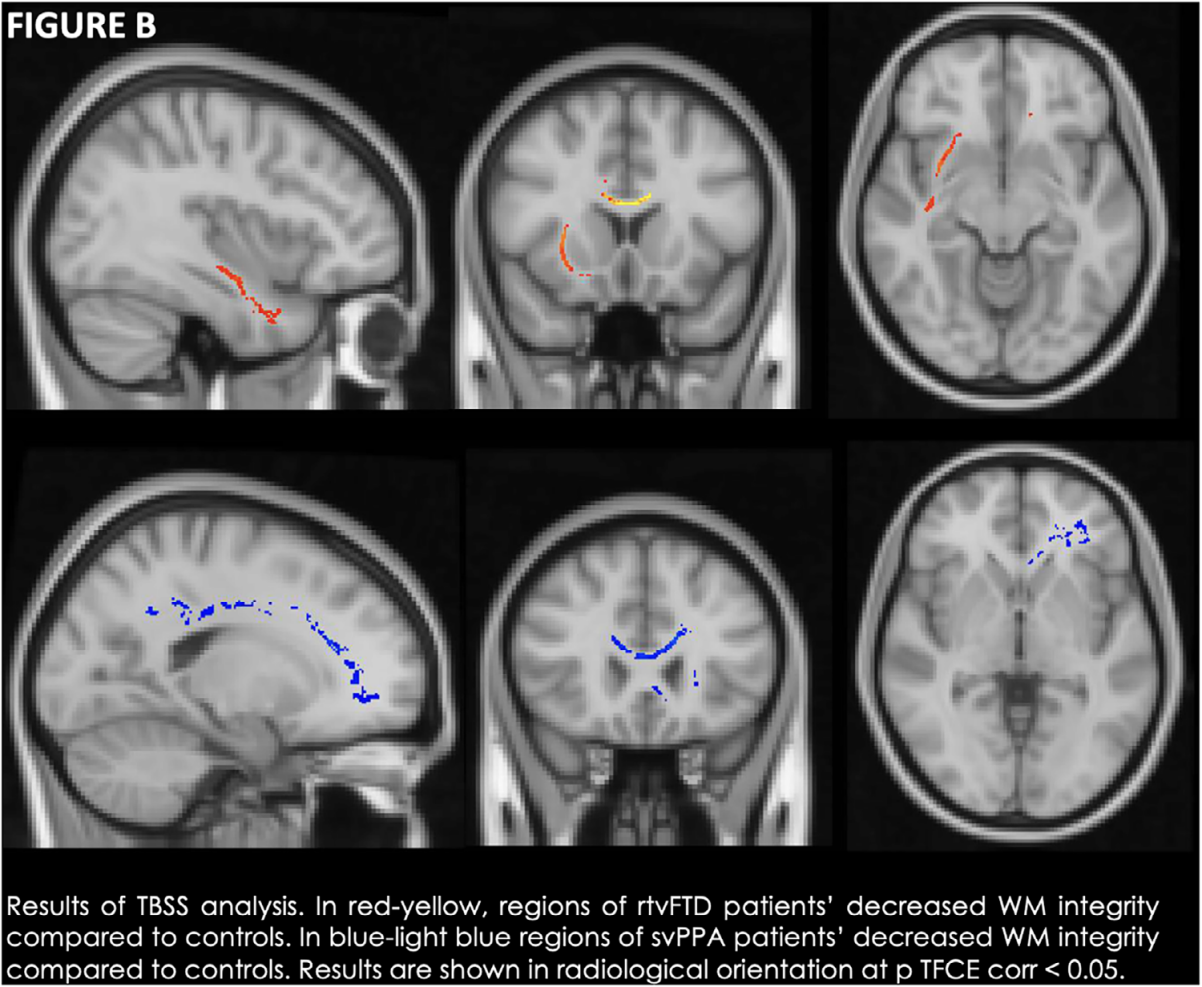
# Right temporal variant frontotemporal dementia: a multimodal MRI analysis
